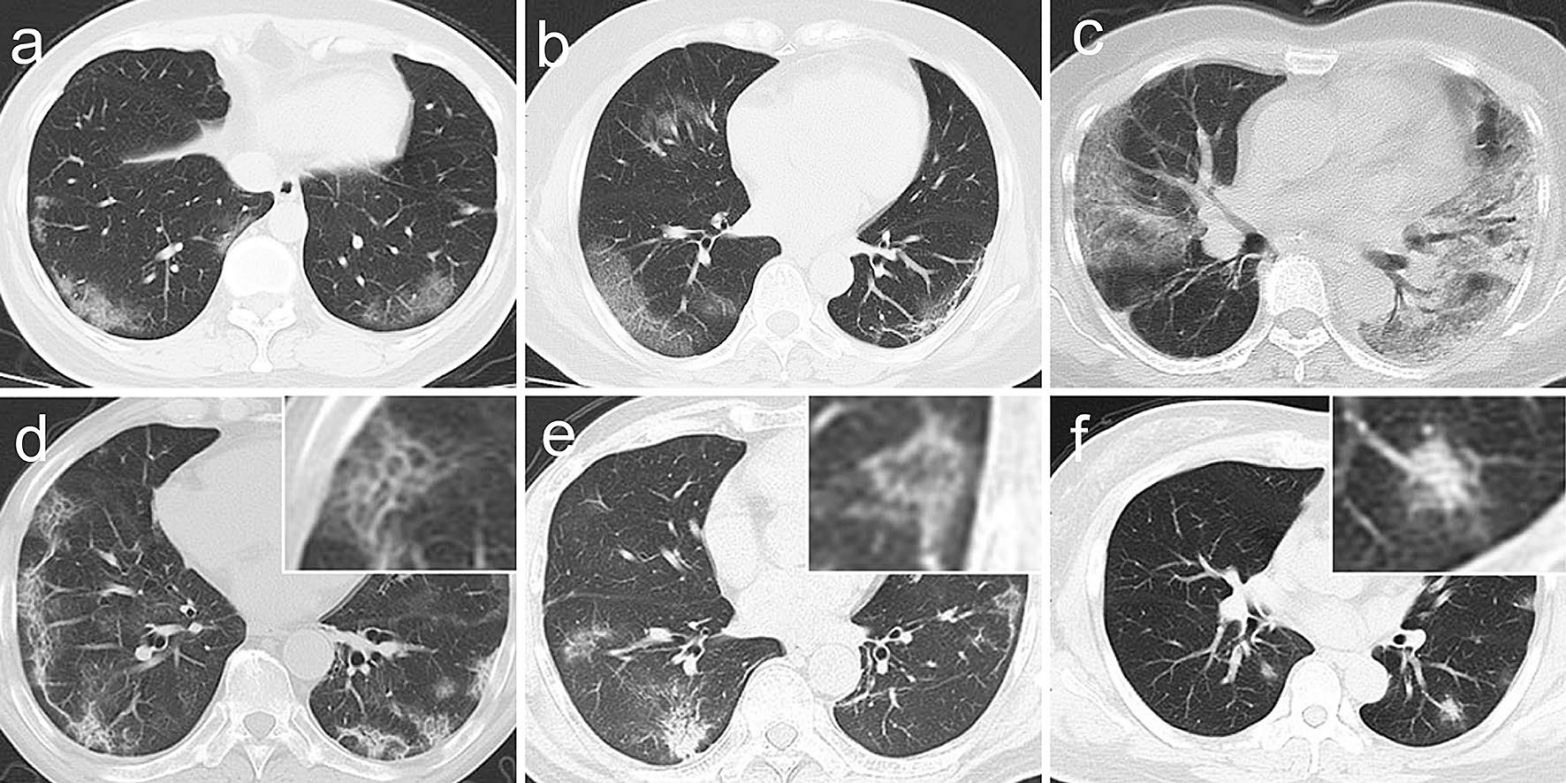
Chest CT findings of COVID-19 pneumonia on transaxial images. (a) Bilateral and peripheral ground-glass opacities in the lower lobe; (b) Ground-glass opacities along peripheral and peribronchovascular distribution in the right lower and middle lobes, subpleural curvilinear line in the left lower lobes; (c) Consolidation with air bronchogram in the left lung; (d) Crazy paving pattern along peripheral distribution in bilateral lungs; (e) Reversed halo sign in the left lower lobe; (f) Halo sign in the left lower lobe.
